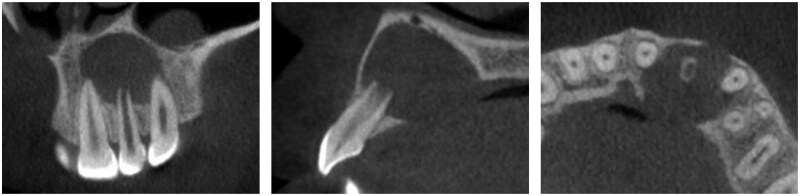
CBCT coronal, sagittal and axial views before surgery.
